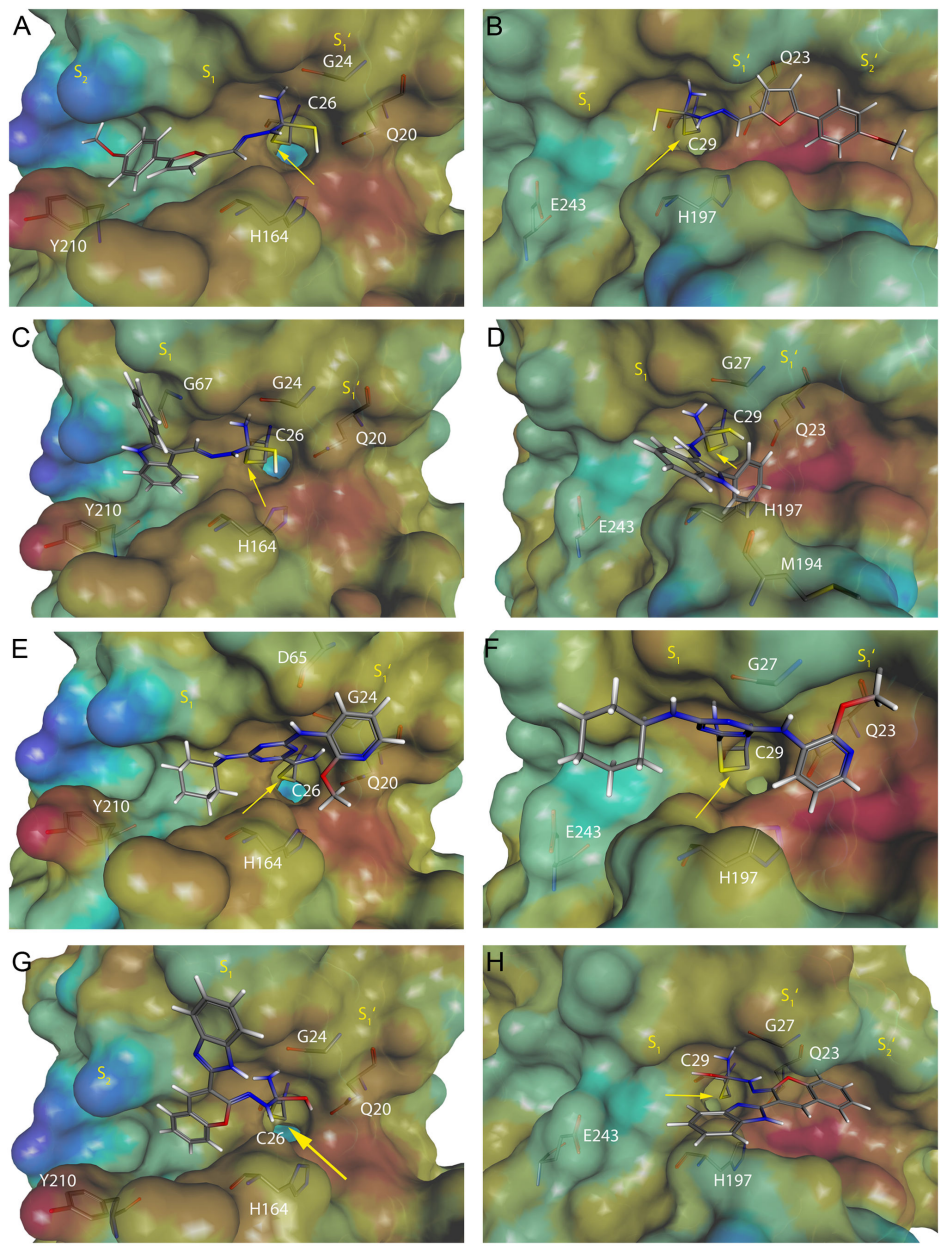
Covalent docking solutions of thiosemicarbazone-, nitrile- and semicarbazone-based inhibitors in the active site of the *L*. *mexicana* CPB2.8∆CTE homology model (A, C, E, G) and the X-ray structure of BtCatB (B, D, F, H). The transparent solvent accessible surface of the active sites is colored by lipophilic potential. The color ramp ranges from brown (highest lipophilic area of the surface) to blue (highest hydrophilic area of the surface). The relevant cysteine residue has been excluded from surface generation for visibility reasons of the covalent bond between the enzyme and the inhibitor molecule. Relevant amino acids of the active sites and the corresponding inhibitors are depicted in capped-stick representation and colored by atom type. The yellow arrow marks the covalent bond between the catalytic cysteine and the inhibitor. A: CP247129 covalently docked into the active site of CPB2.8∆CTE, B: CP247129 covalently docked into the active site of BtCatB, C: CP247128 covalently docked into the active site of CPB2.8∆CTE, D: CP247128 covalently docked into the active site of BtCatB, E: CP241026 covalently docked into the active site of CPB2.8∆CTE, F: CP241026 covalently docked into the active site of BtCatB, G: CP229988 covalently docked into the active site of CPB2.8∆CTE, H: CP229988 covalently docked into the active site of BtCatB.
